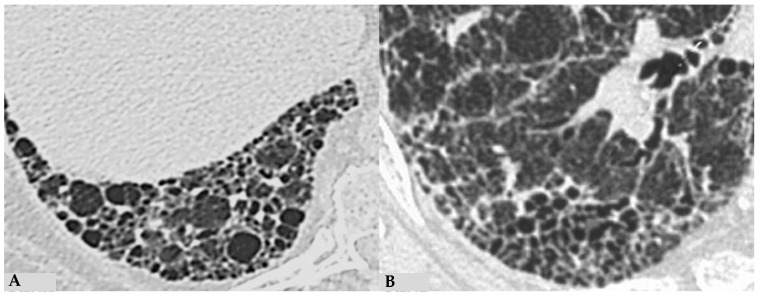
End-stage lung: HRCT magnification of right lower lobes in two different patients. Profuse honeycombing in chronic hypersensitivity pneumonitis (HP) with evidence of multiple multilayered pulmonary cysts ranging in size from a few mm to 10 mm (A); honeycombing in idiopathic pulmonary fibrosis (IPF) characterized by minute clustered pulmonary cysts coexisting with subpleural distribution of traction bronchiectasis and bronchiolectasis (B).
